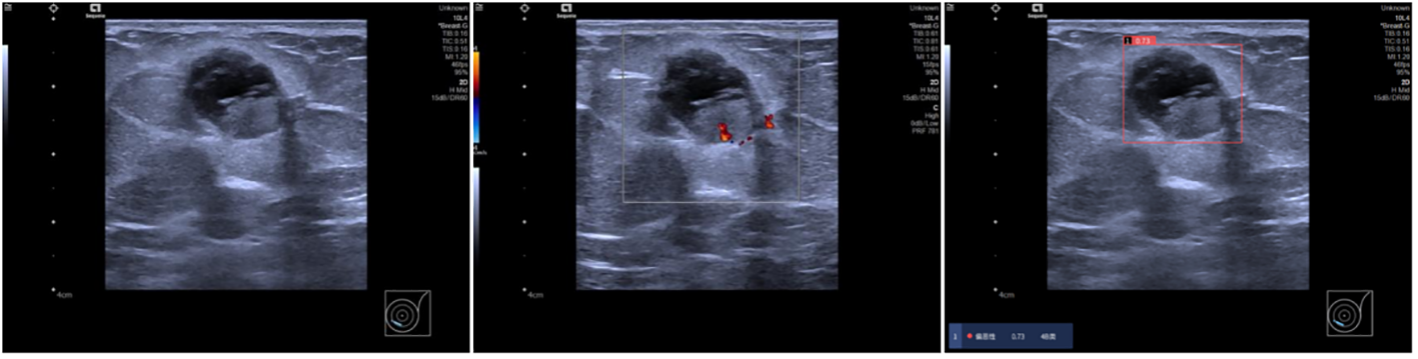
Ultrasound findings in a 69-year-old female patient showing solid cystic masses at 7-8 o’clock positions in the left breast with clear boundaries, intact envelopes, and punctate blood flow signals. Radiologists categorized the lesions as BI-RADS 4a, while AI diagnosed them as BI-RADS 4b with a malignant probability value of 0.73. Pathology confirmed left breast sternal papillary carcinoma and partial invasive carcinoma.
